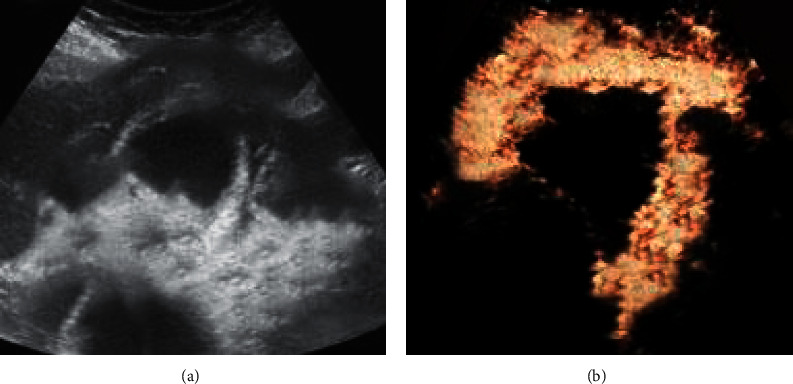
Contrast ultrasound image: (a) two-dimensional ultrasound image of the cross section of the gallbladder; (b) contrast-enhanced ultrasound.
